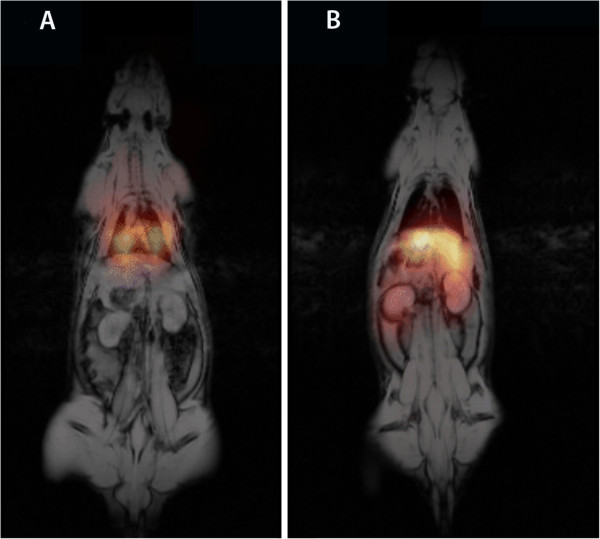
Multimodality imaging using SPECT/MRI. SPECT enables the functional visualization of MBs in the lungs 1 h post injection (A) and in the liver and kidneys 24 h post injection (B), while MRI enables the visualization of an anatomical image and SPION in the liver post injection and 24 h post injection (A and B).
